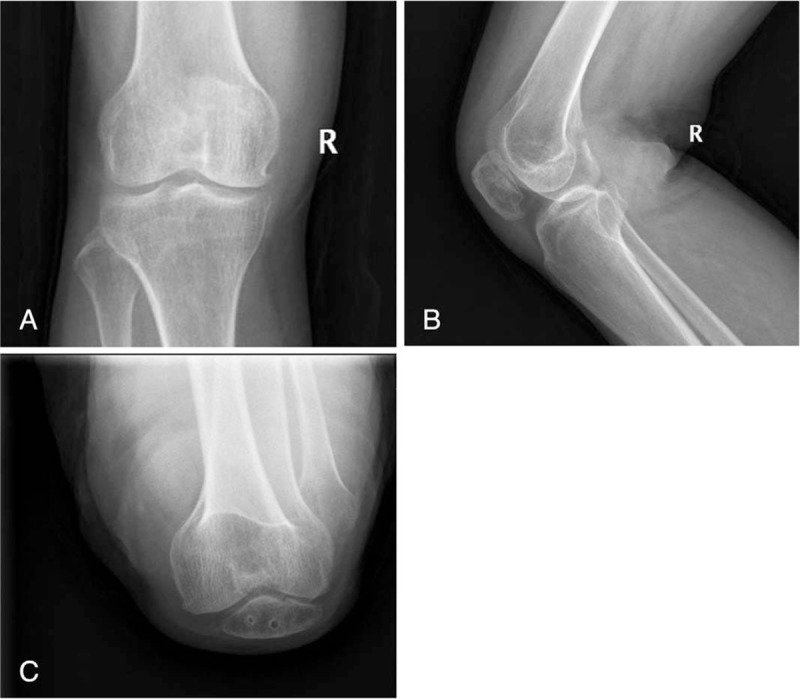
Radiographs after implant removal showing osseous healing of the fragments (A–C).
